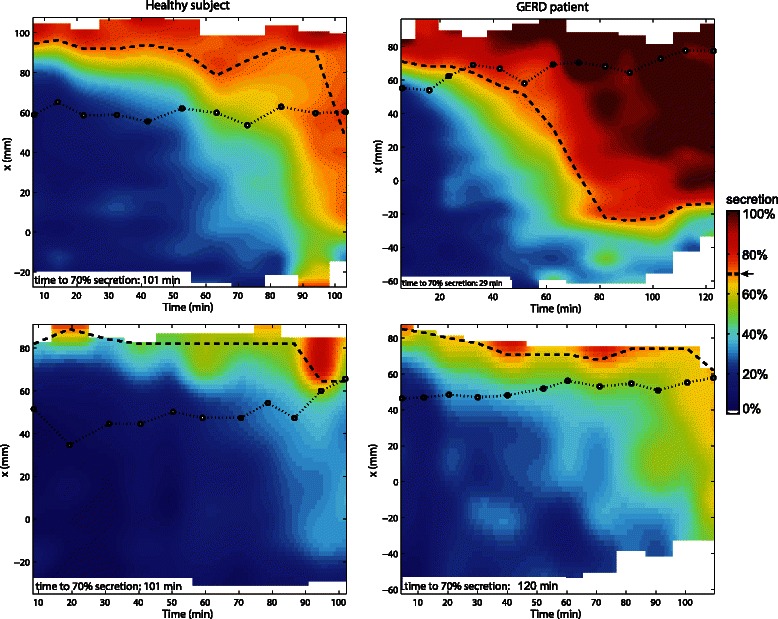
Layer graphs including EGJ position and time to Layer formation. Layer graphs of a healthy subject (left) and a GERD patient (right) under placebo (top) and PPI therapy (bottom). The vertical axis represents the MR image x-axis, which is aligned along the direction of gravity. The colours code the average *%secretion*. The threshold level of the layer (i.e. ≥70 % secretion) and the position of the EGJ are indicated as white dashed and black dotted line, respectively
